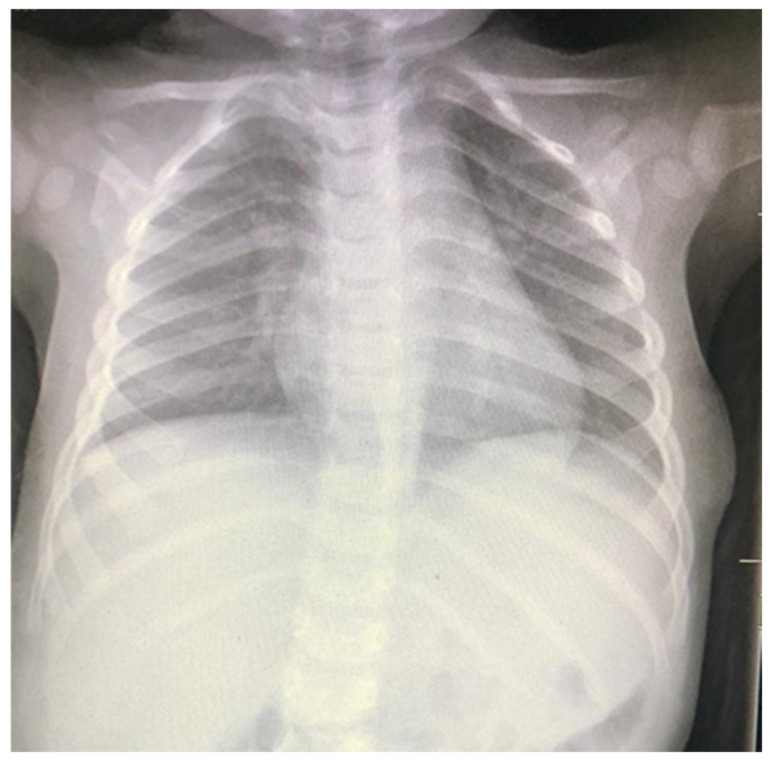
Chest X-ray showing the soft tissue mass with intact ribs.
